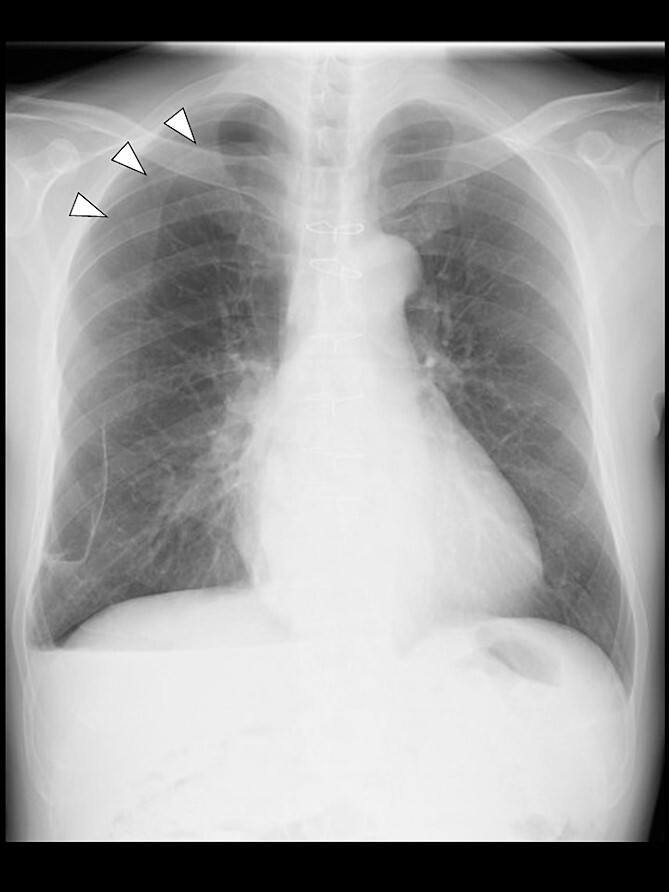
A chest radiograph in the upright position on POD 3 showing a residual right pneumothorax (arrowheads).
